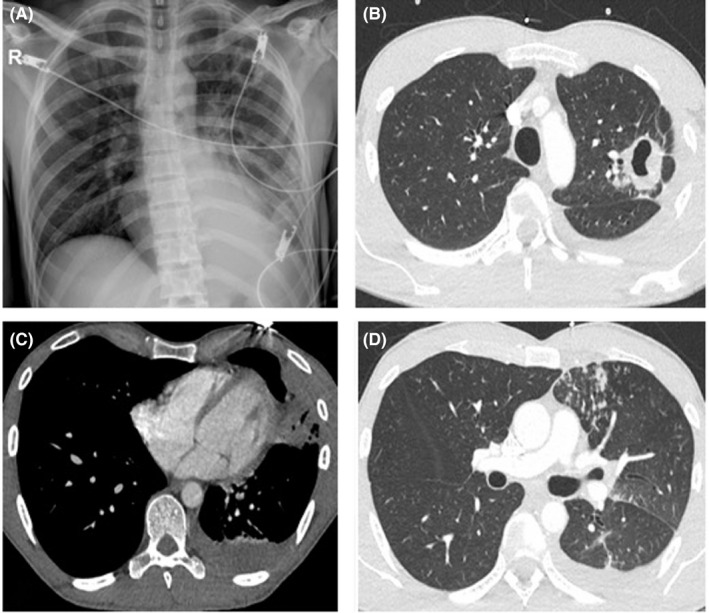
A, Chest X‐ray showing a cavitated lesion with thick edges at the left pulmonary apex associated with infiltrates with alveolar occupation in the left lower lobe. B‐D, Computed tomography showing the left apical cavity with irregular edges with hydro air level associated with inflammatory‐appearing nodules, ipsilateral endobronchial dissemination, and left pleural effusion
